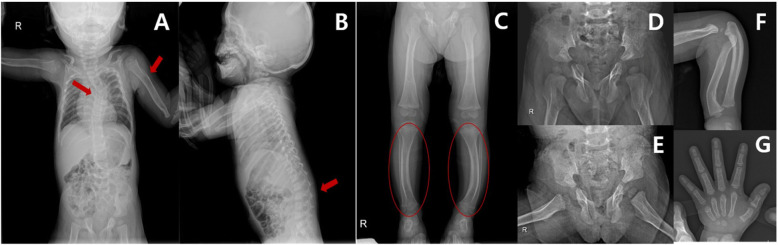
Plain radiographs of the patient at age 16 months. Overall, the patient’s bones were curved and thin. Bone age was not delayed, but her bones did not have normal alignment or cortical bone maturity and showed osteodysplasia. a, b, red arrow The patient had a thoracolumbar kyphoscoliosis with a humeral cortical irregularity and thin, wavy ribs. c, red circle Bilateral bowed leg deformities with Erlenmeyer flask deformity were observed. d, e The pubis and ischium were hypoplastic, and coxa valga was present bilaterally. f The ulna and radius were curved. g Bone age was normal, according to the hand radiographs
